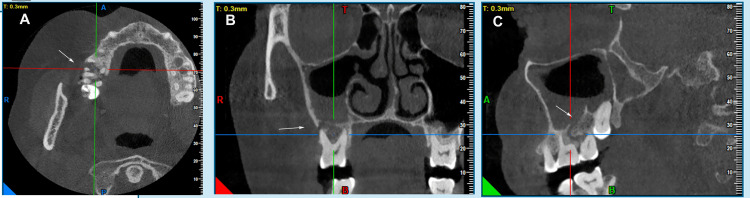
Cone-beam computed tomography (CBCT) of the maxilla showing opacification in the region of the second molar and a soft tissue shadow in the maxillary sinus on the right side: (A) axial view; (B) coronal view; (C) sagittal view. Original images of the patient, used with the patient's permission.
